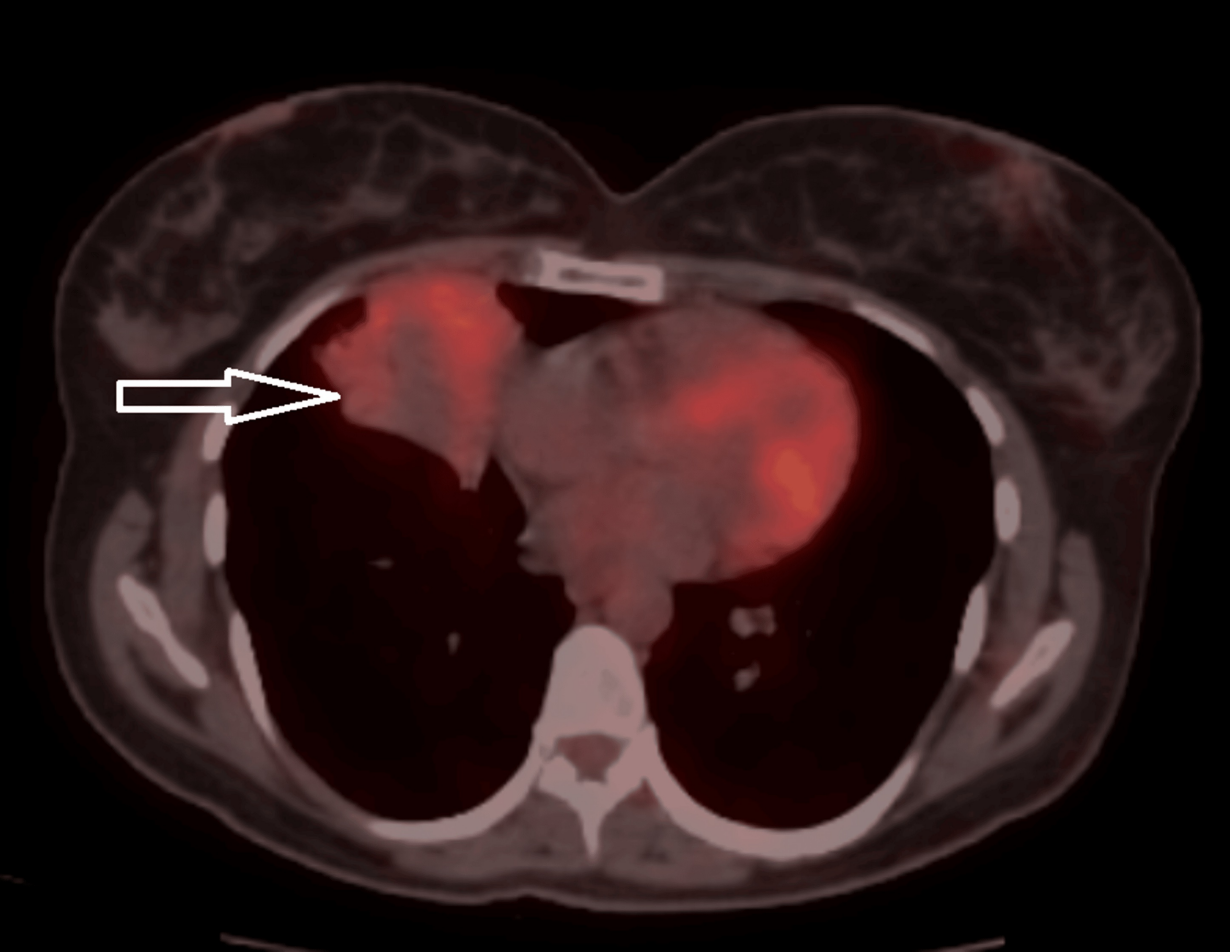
PET-CT revealed a mass in the right middle lobe with a hypometabolic center and moderate fluorodeoxyglucose uptake.
